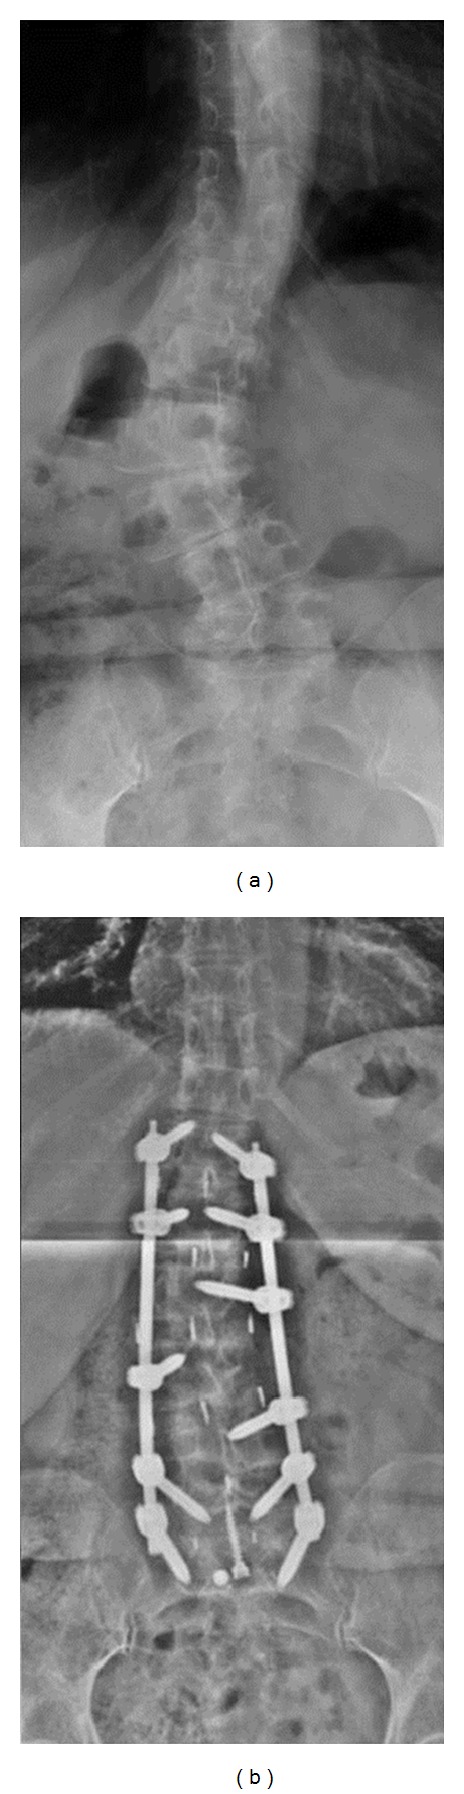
Preoperative (a) and postoperative (b) anteroposterior radiographs of the lumbar spine in a patient treated with XLIF with percutaneous pedicle screws and rods.
